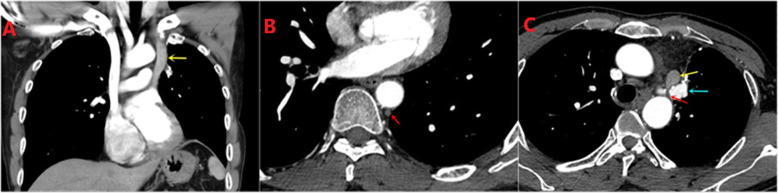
Persistent left superior vena cava(PLSVC) and left azygos vein (Chest CT which was shot 8 months after surgery). The left azygos vein is not easy to be detected before surgery.(red arrow: left azygos vein, yellow arrow: PLSVC, blue arrow: left pulmonary artery trunk)
